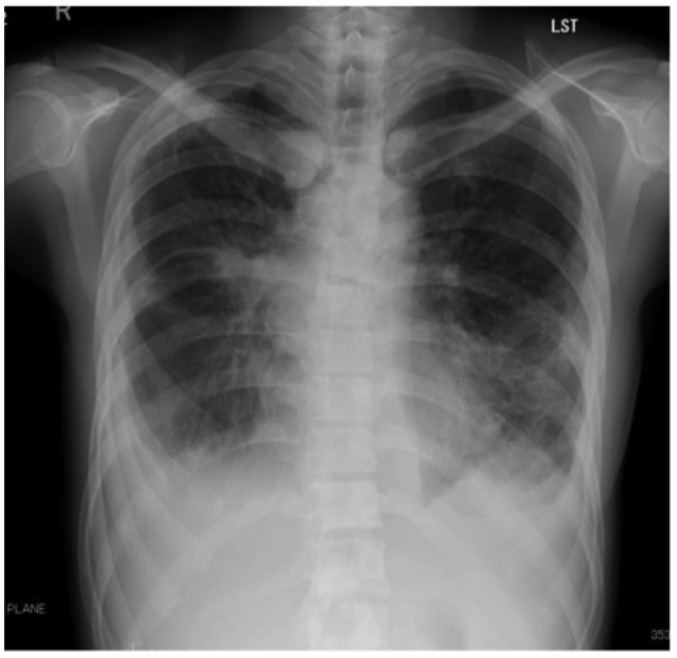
Chest X-ray scan revealed a tumor shadow in the right hilar area, an invasive shadow in the left middle and lower lung fields, and pleural effusion on both sides.
